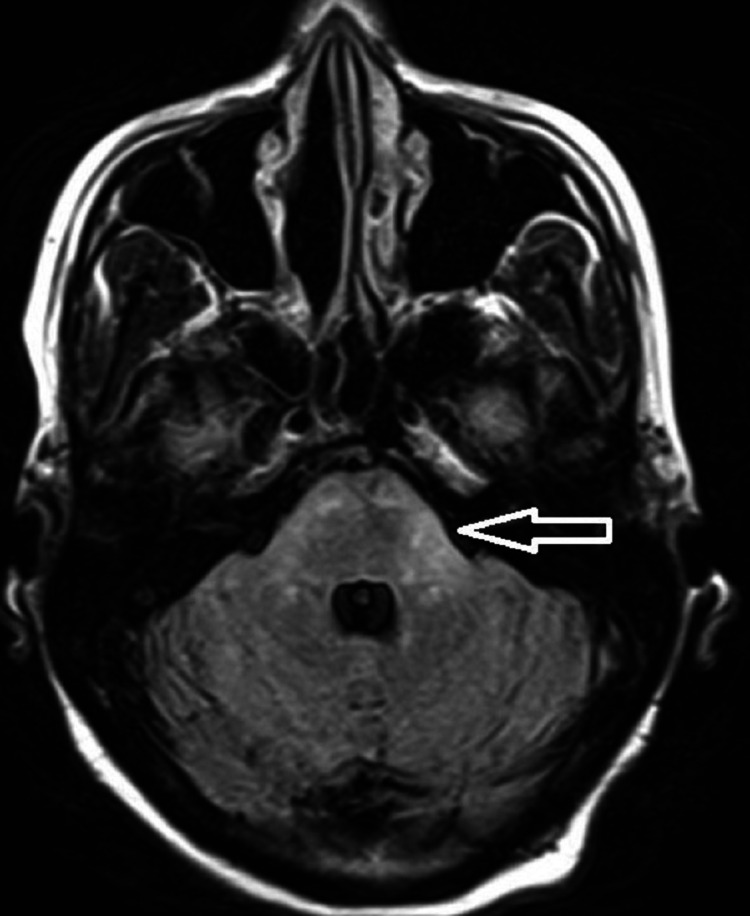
FLAIR image with an arrow showing hyperintense signal abnormality over left brachium pontis FLAIR: Fluid-attenuated inversion recovery
